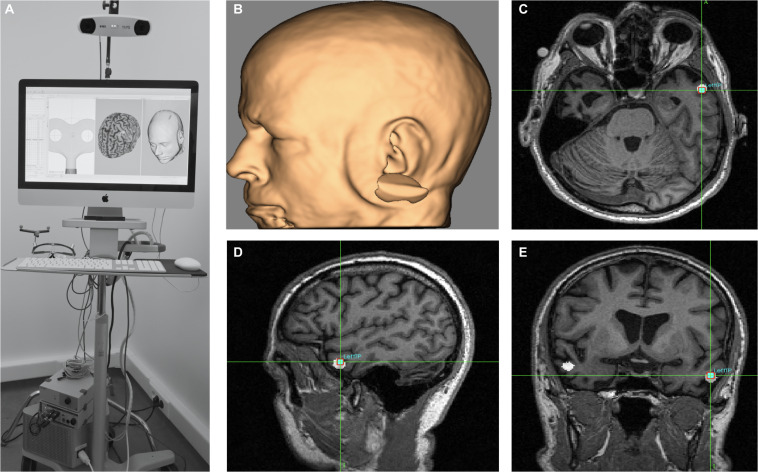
MRI-based frameless neuronavigation system used to place the stimulation devices (rTMS coil or tDCS electrodes) in optimal scalp location, overlying with the shortest-path a given cortical target. To this end, (A) a high resolution T1-3D MRI volume is obtained. Then cortical targets are labeled either on the basis of anatomical landmarks or by targeting MNI/Talairach coordinates (see white 5 mm radius spheres in panels c, d and e on the left and right anterior temporal lobe, ATL). (B) 3D reconstruction of the patient’s head surface based on his individual T13D MRI sequence. Panels (C–E) show axial, sagittal and coronal sections of the ATL target (see crosshairs) placed on MNI coordinates (*x* = -52, *y* = 2, *z* = -28) in a sv-PPA patient prior to an anodal tDCS treatment (as in Teichmann et al., 2016). The MRI based neuronavigation systems allow to plan pre-hoc the optimal scalp site for tDCS electrodes or site, orientation, angulation and tilting of the TMS coil.
